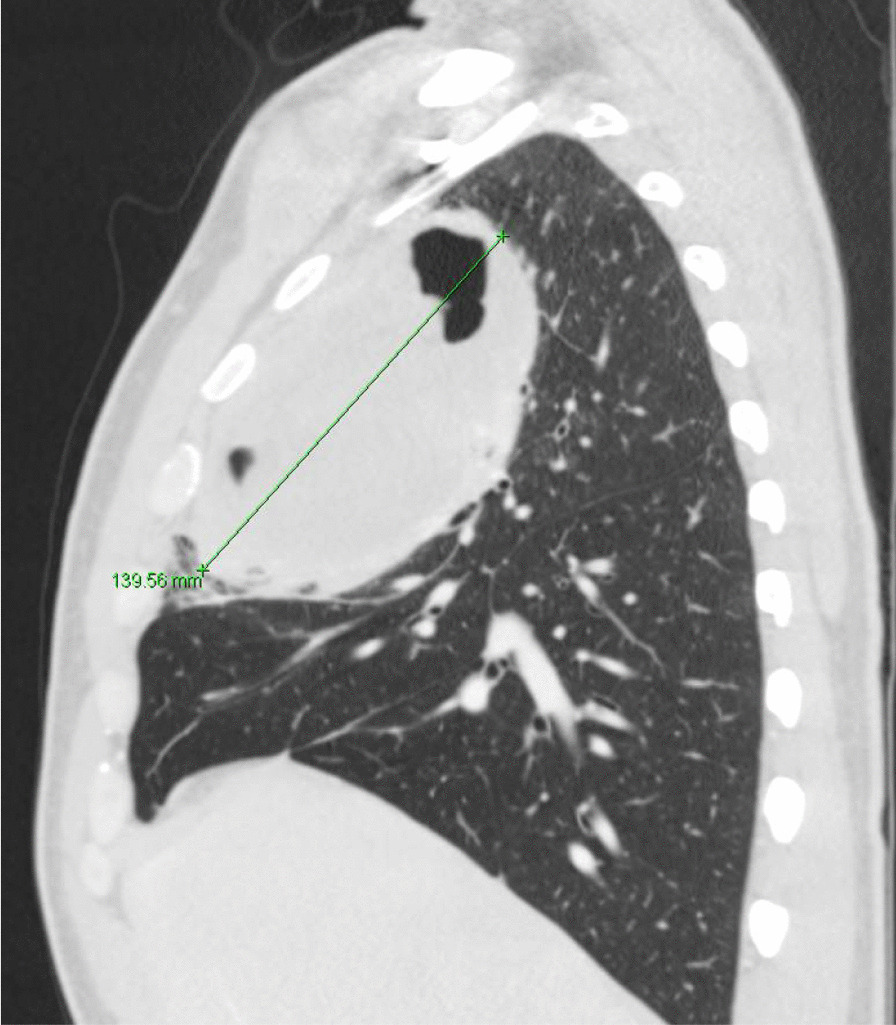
Initial CT chest with contrast (sagittal image). Right upper lobe cavitary lesion
